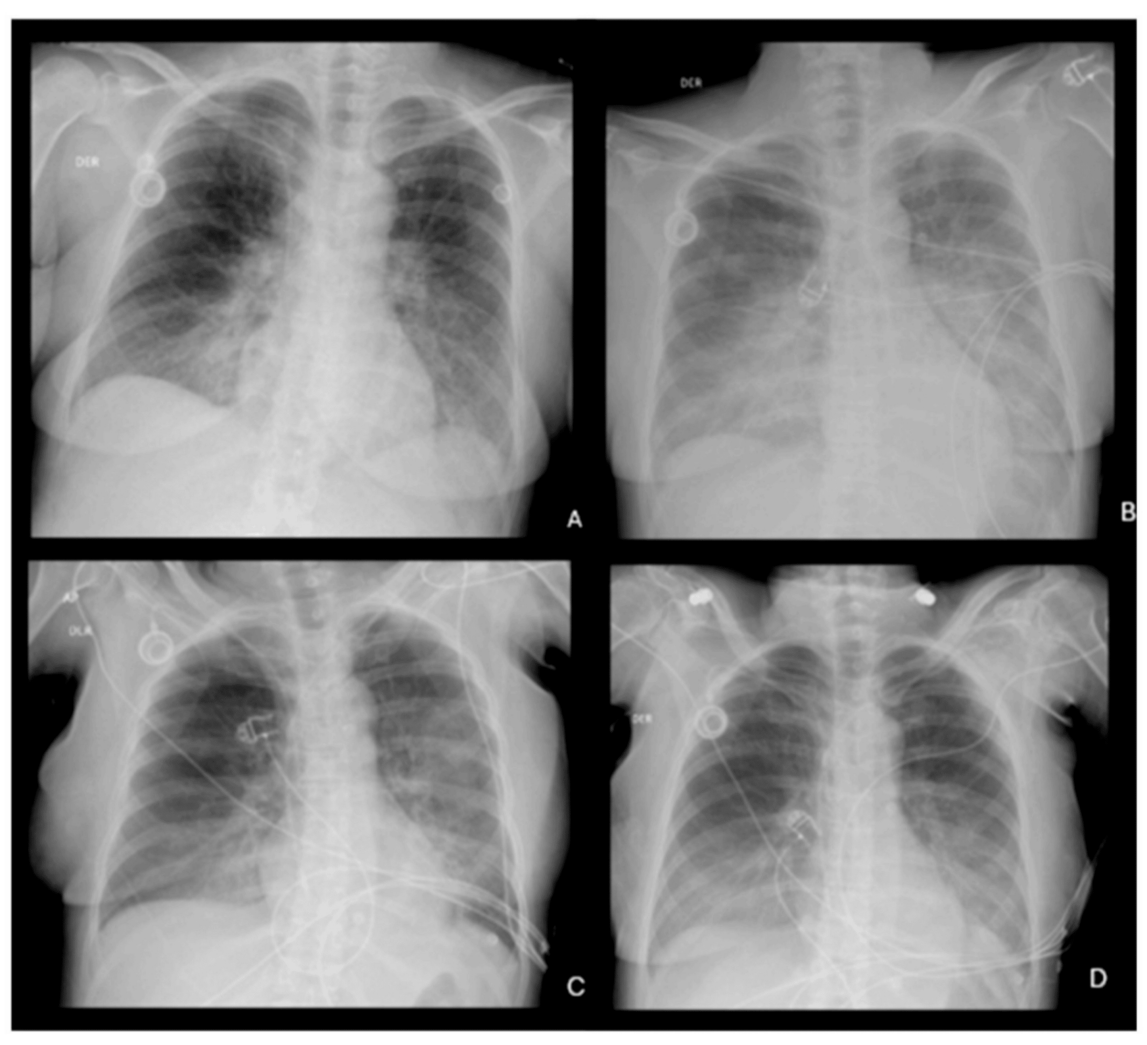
Chest X-ray shows bilateral pulmonary congestion with progressive worsening compared to the previous day. (A) Chest X-ray at admission before transfer to the intensive care unit (ICU) (day one). (B) ICU admission with signs of bilateral pulmonary congestion (day two). (C) Radiological improvement after starting levosimendan (day three). (D) Progressive resolution of congestion with 12 mL/hour of levosimendan and support with norepinephrine (day four).
